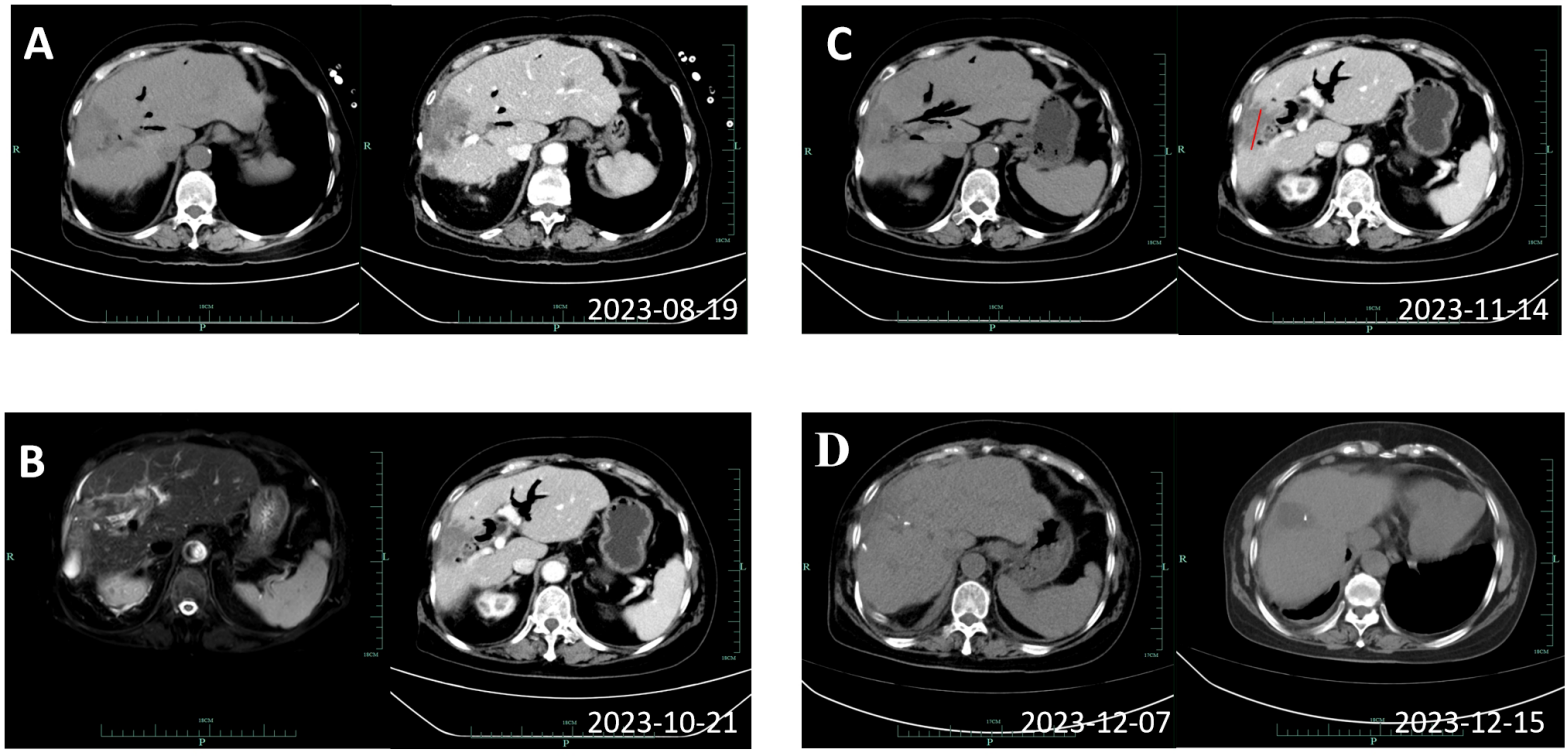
Imaging review results of the patient during systemic therapy. (A) Showing enhanced CT results of 2023-08-19, before the third cycle. (B) Showing magnetic resonance imaging and cholangiopancreatography results of 2023-10-21, before the sixth cycle. (C) Showing enhanced CT of 2023-11-14, after 6 cycles with a reduction in the longest diameter of the tumor to 4.9cm. (D) Display the results of the enhanced CT scans of the two postoperative reviews.
